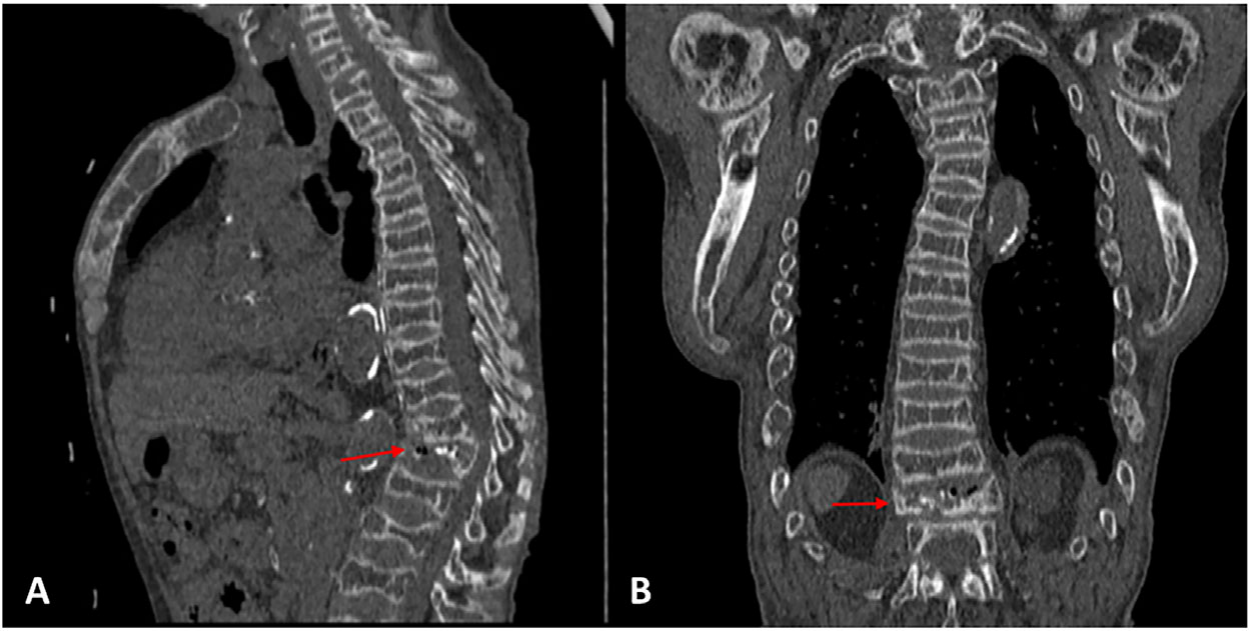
Noncontrast CT scan of the spine. Multiple thoracic and lumbar vertebrae compression fracture and multiple pyramidal instability . (A) Sagittal reformation showed kyphosis and T_12_ wedge deformity (red arrow). (B) Coronal reformation showed T_12_ compression fracture (red arrow).
